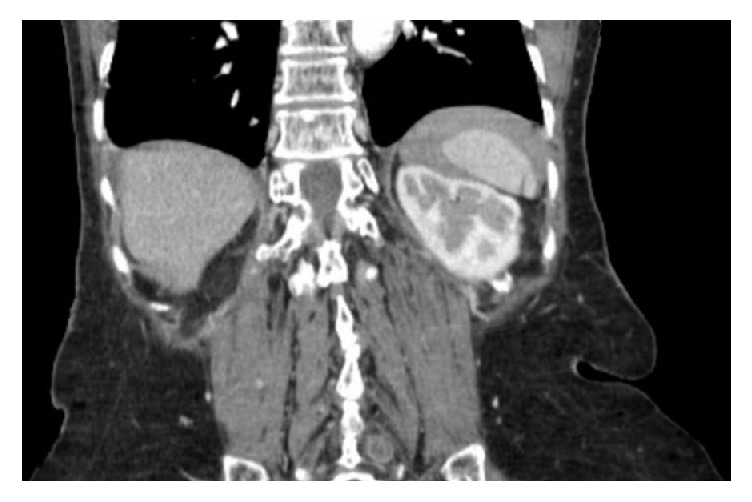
Repeat CT of abdomen and pelvis with contrast revealing an apparent linear defect measuring 0.9 cm involving the posteroinferior margin of the spleen. Again seen, consistent with prior study is the presence of a moderate amount of hyperdense free intraperitoneal fluid, indicative of hemoperitoneum.
